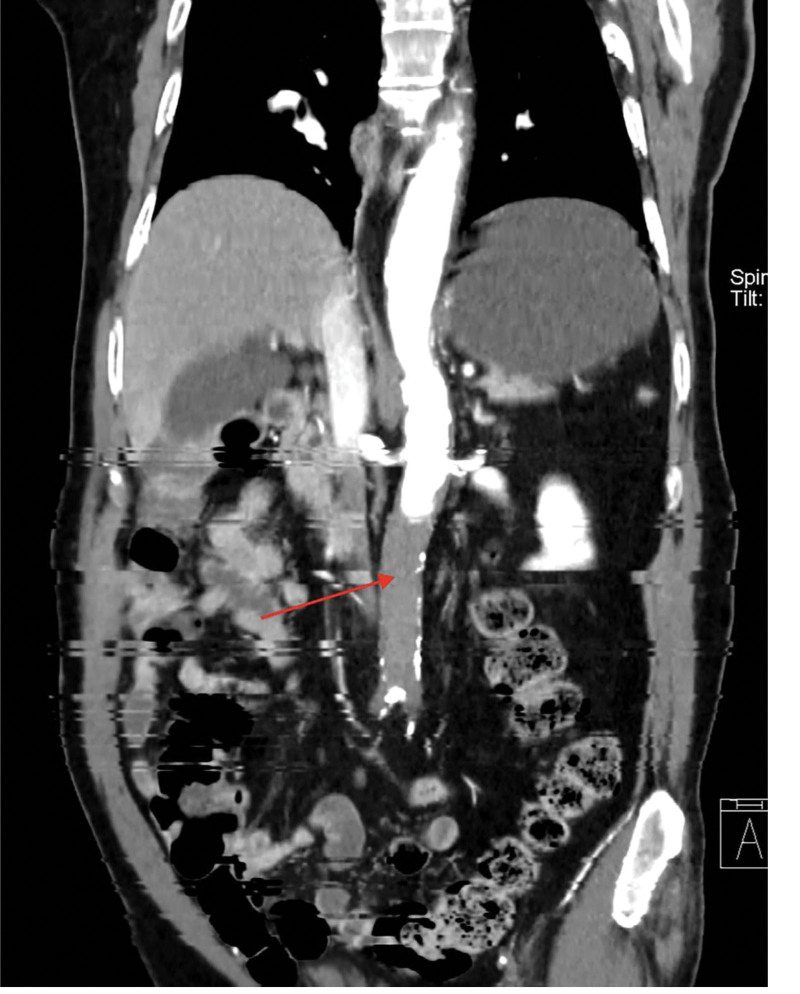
Computed tomography angiography of the chest and abdomen (coronal view) demonstrates occlusion of the abdominal aorta (below the level of the renal artery origins).
